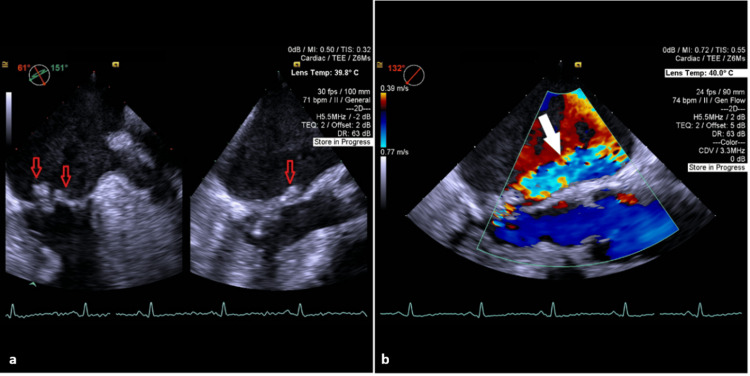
Transesophageal echocardiographic views of mitral valve vegetations and regurgitation (A, B). A - Biplanar projection and visualized vegetations in the area of the posterior leaflet of the mitral valve (indicated by red arrows), B - Transesophageal echo and modified long axis view
